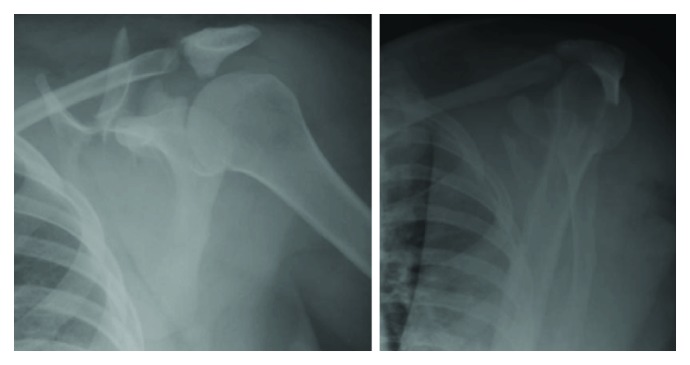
AP and lateral radiographic views of the scapula showing a comminuted and displaced fracture of the scapular spine and body with an avulsion of the acromion base.
